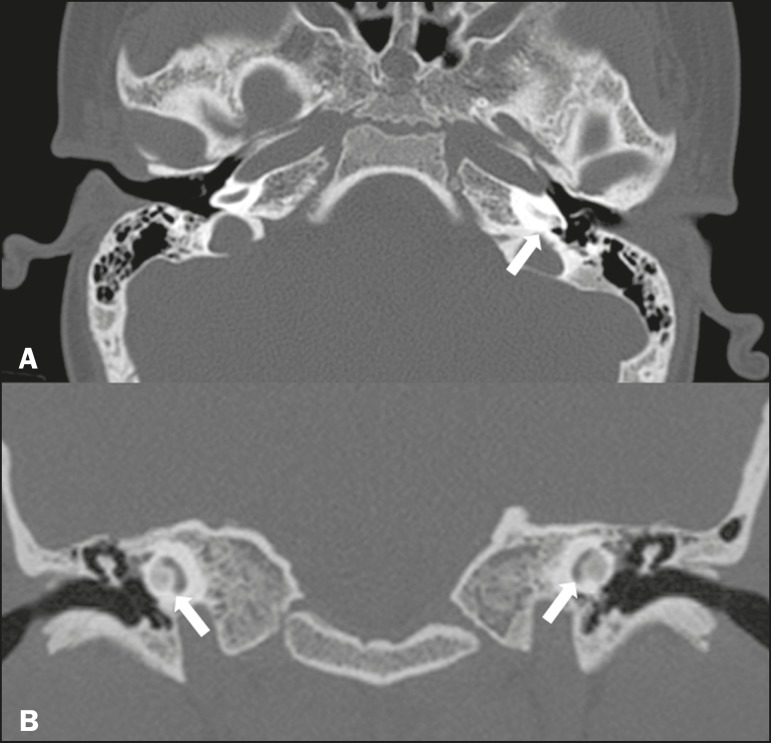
Labyrinthitis ossificans. A: Axial CT scan, with bone
